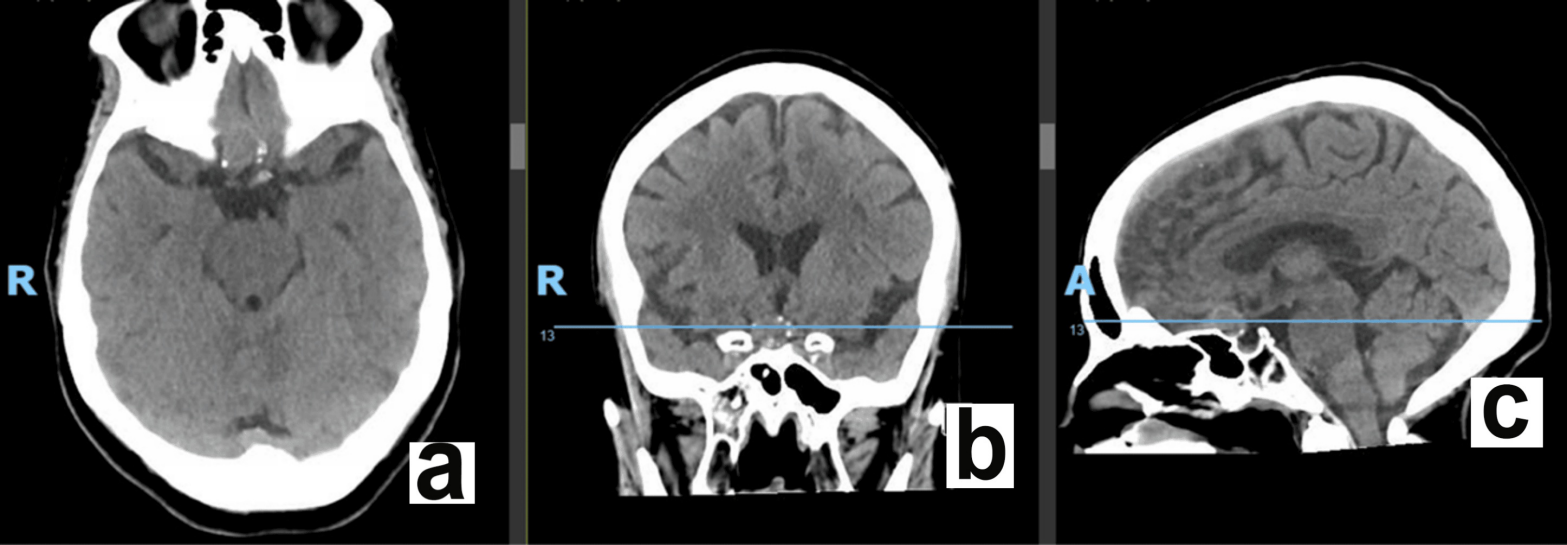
Preoperative axial (a), coronal (b), and sagittal (c) head CT scans. Masslike abnormality just anterior to the sella near midline measuring 1.4 x 1.0 x 1.4 cm.
